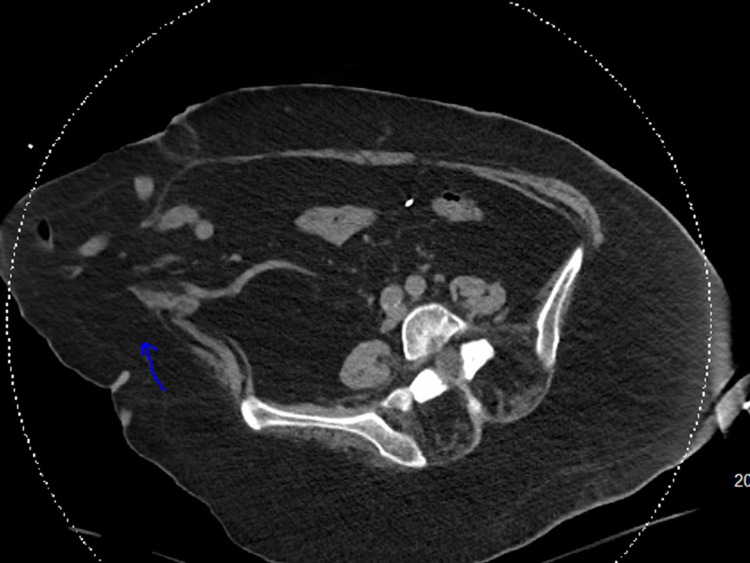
CT abdomen showing large right paramedian ventral hernia sac that contains abdominal contents (blue arrow).
